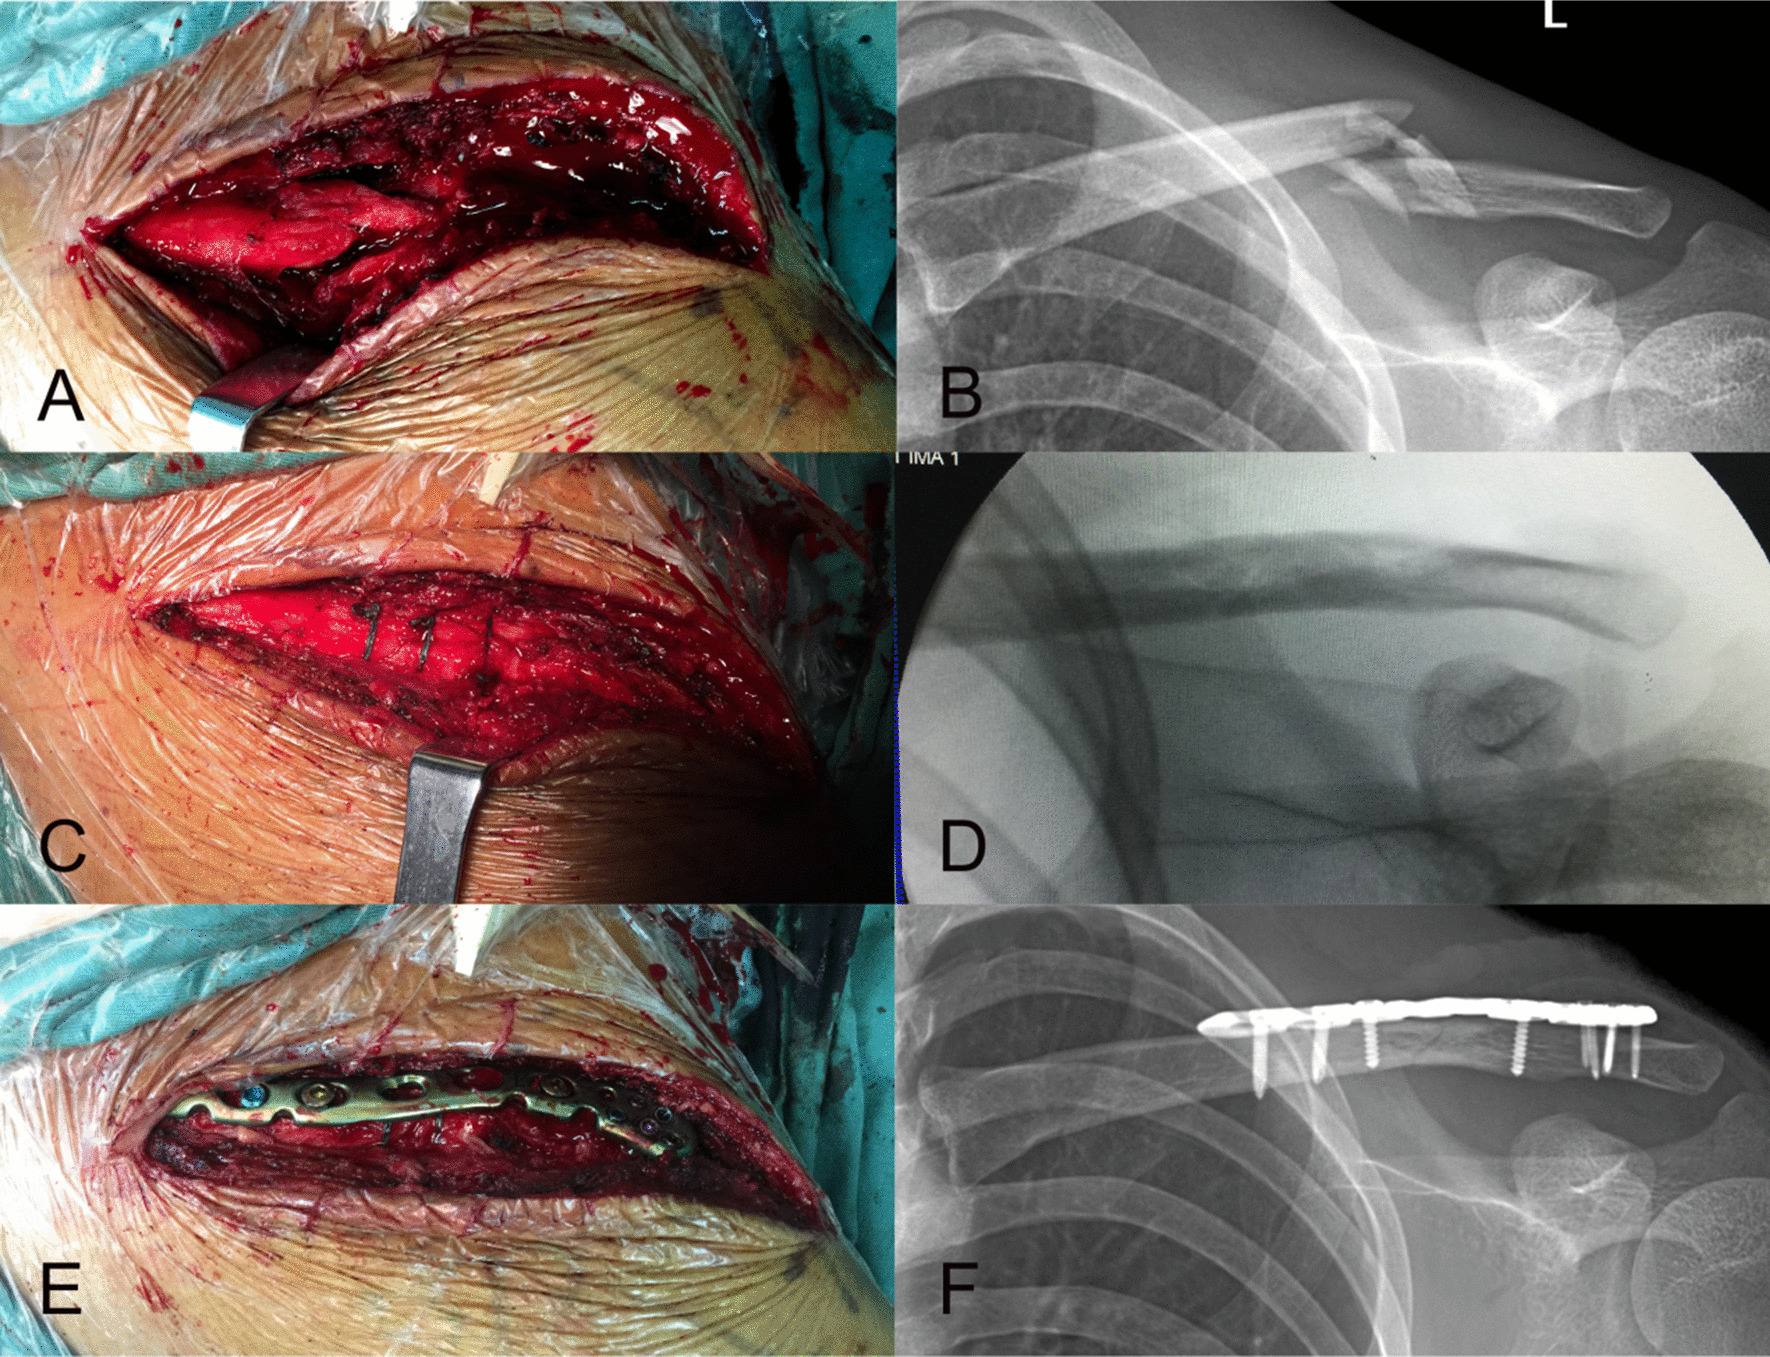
Intraoperative digital image and X-ray film of the clavicle fracture fixation. A Exposure of comminuted midshaft clavicle fracture. Many displaced bone fragments could be found within the fracture region, and the soft tissue attached to the bone fragments was maximally preserved. B Preoperative X-ray film of the clavicle. C Doubled-suture Nice knot fixed the fracture in anatomical reduction. D Intraoperative X-ray film revealed that the fracture achieved anatomical reduction after the clavicle was fixed with doubled-suture Nice knot. E A locking plate was subsequently applied to fix the fracture. F Postoperative X-ray film of the clavicle
